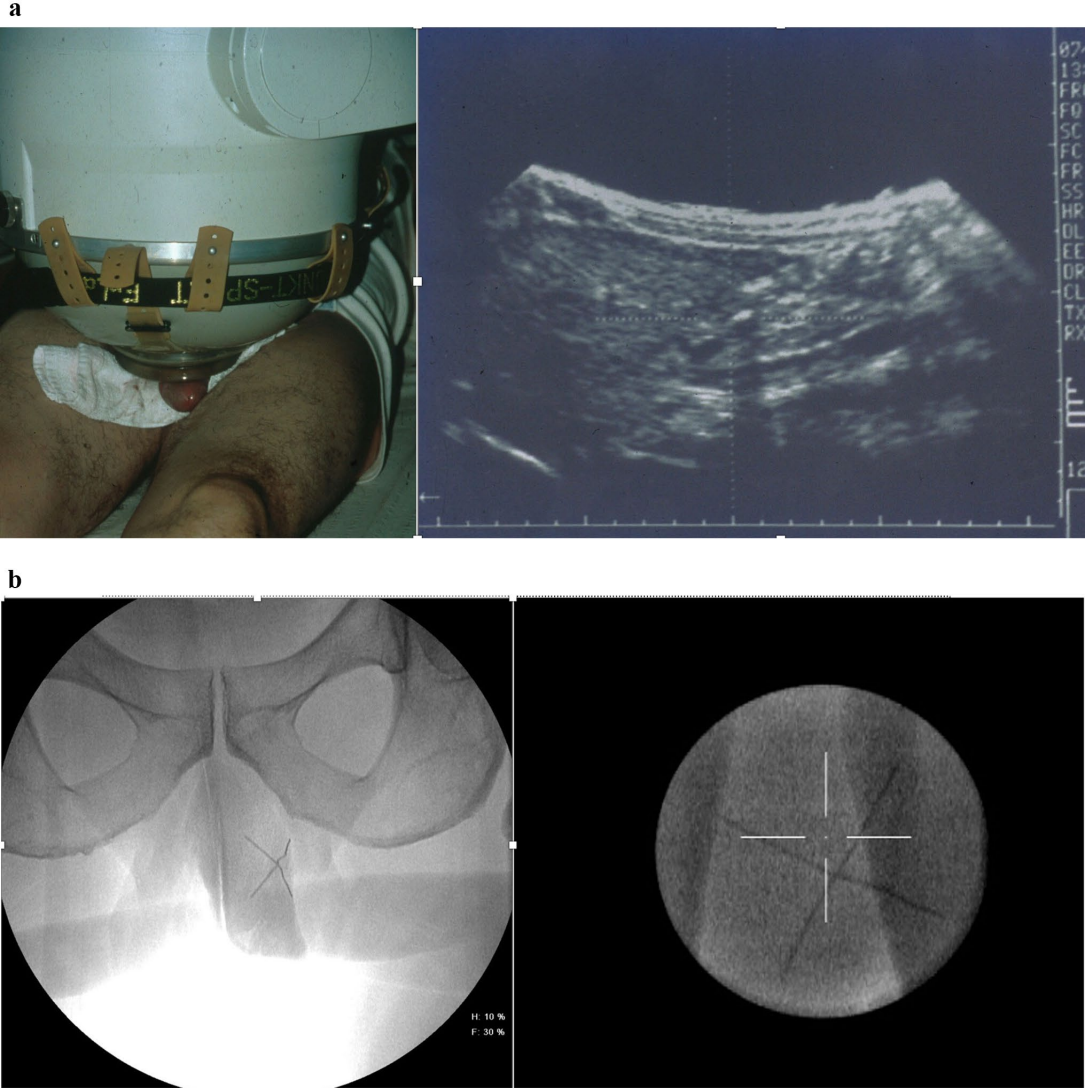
High-intensity extracorporeal shock wave therapy (ESWT) for management of Peyronie’s disease. Treatment technique. a Use of Siemens Lithostar Overhead Module with coupling device for extracorporeal shock wave lithotripsy in children. Ultrasonic localization of plaque. 21 kV, 3000–4000 impulses, level 6, 0.5 mJ/mm^2^, 1 Hz. b Use of Siemens Lithoskop with puncture of the plaque by two crossing needles (left) and in-line fluoroscopic localization (right)
